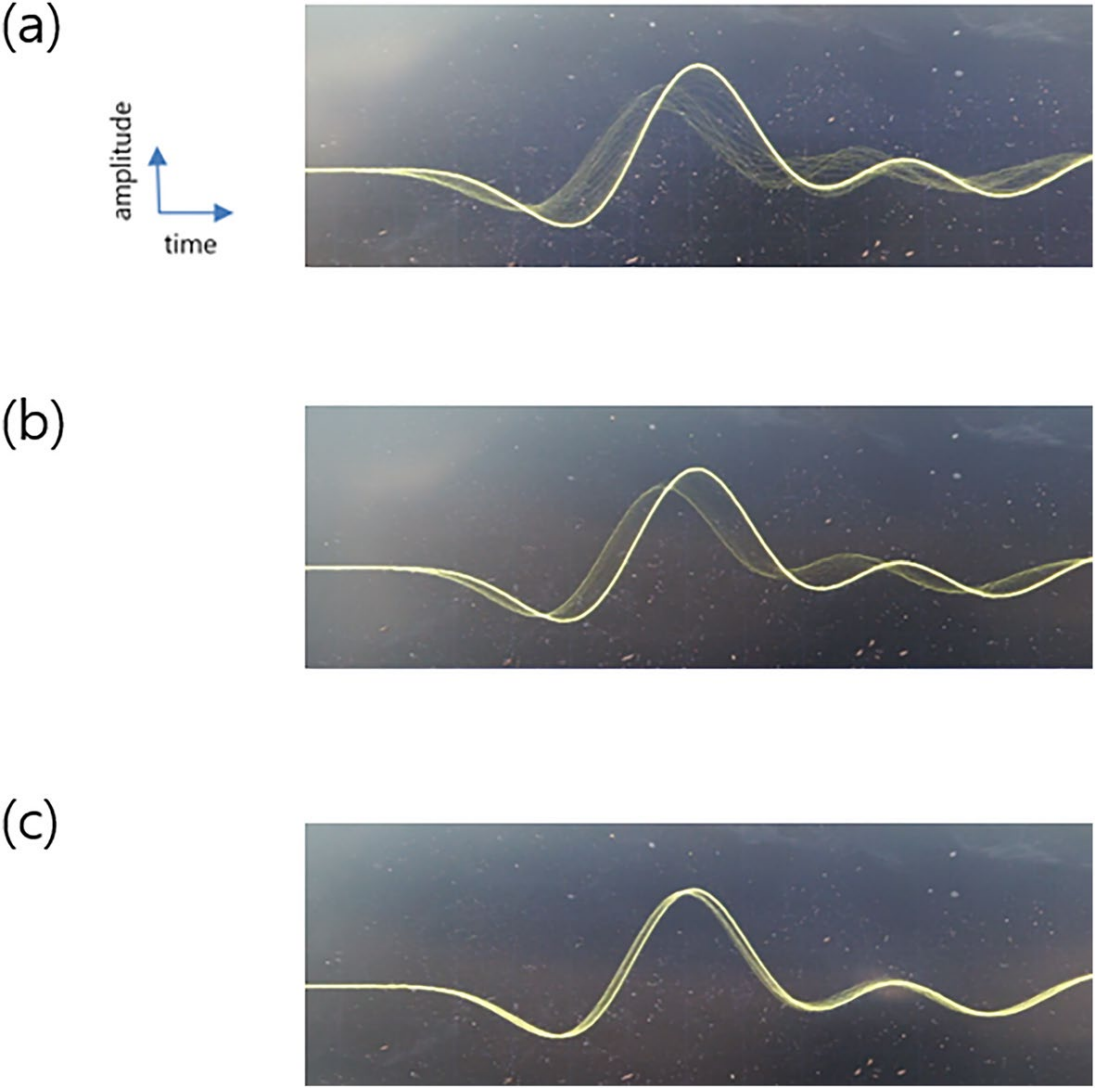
Ultrasonic signals at different LPMs. (A) 1 LPM, (B) 2 LPM, and (C) 4 LPM.
